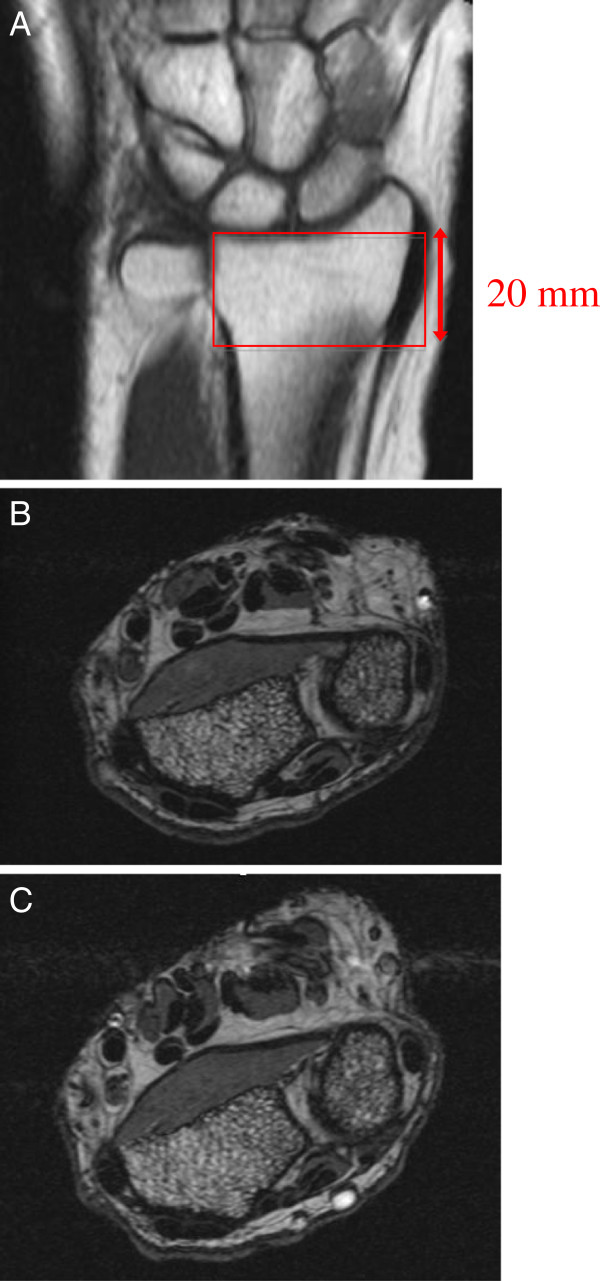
Representative coronal MRI scout scan depicting selection of region of interest for axial slices (A) and matched baseline (B) and follow-up (C) axial MRI images used for analysis.
